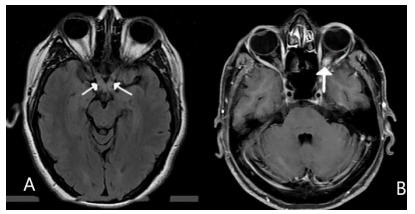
(A) Magnetic resonance imaging in Fluid-Attenuated Inversion Recovery sequence with hyperintensity in both optic nerves in the chiasmatic region. (B) Sequence in T1 with gadolinium with reinforcement of intraocular part of left optic nerve.
